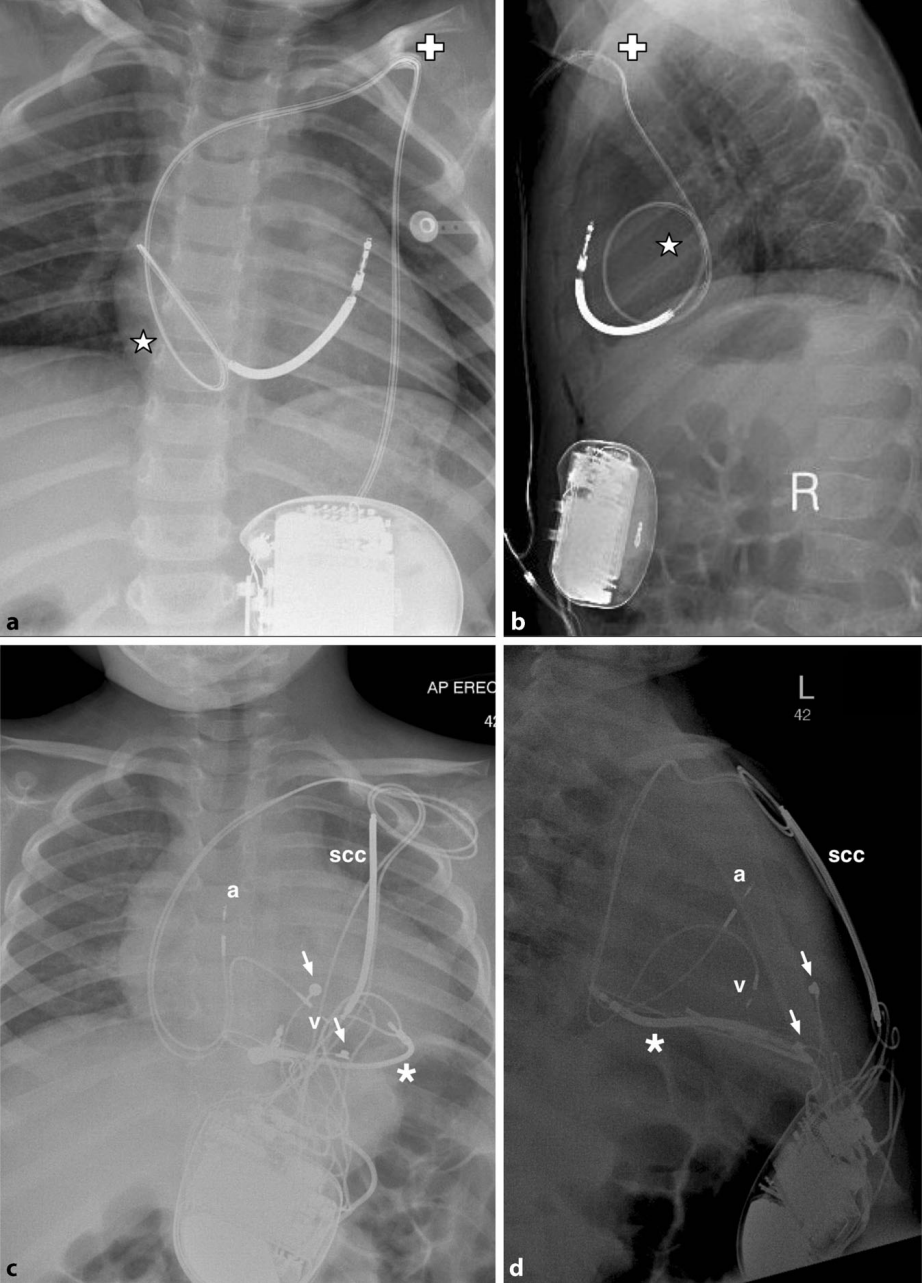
a Posteroanterior and b lateral radiographs demonstrating transvenous pacing system in an 18 kg child with long QT (LQT) syndrome. Generator has been placed abdominally with lead tunnelled to left subclavian vein (cross). Note redundant loop to accommodate growth (star). c Posteroanterior and d lateral radiographs demonstrating a more complex hybrid system in a 15 kg child with LQT type 3. The first ICD implanted was non-transvenous, with intrapericardial coil (asterisk) and epicardial sense and pacing leads (white arrows). Subsequent failure of the epicardial pacing leads with R‑wave undersensing and rising defibrillation threshold necessitated upgrade of the system with subcutanous coil (scc) and transvenous atrial (a) and ventricular (v) pace and sense leads (c and d courtesy of Jasveer Mangat, Great Ormond Street Hospital, London)
